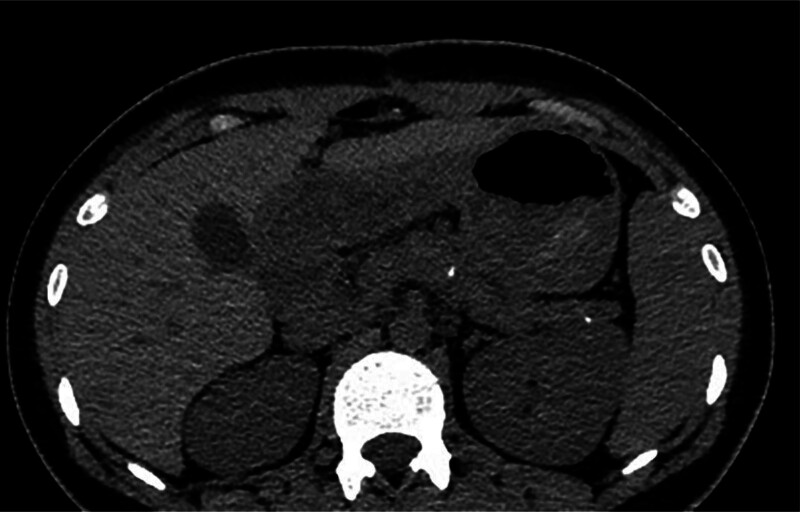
CT reexamination 1 year after surgery. CT = computed tomography.
